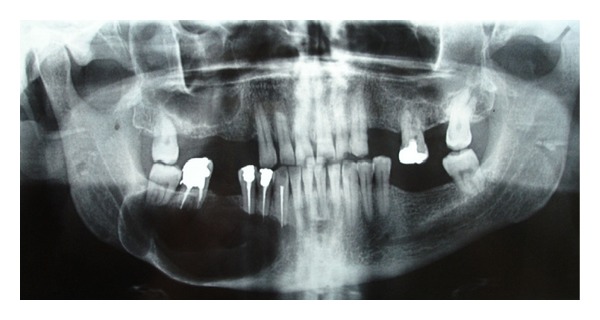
Panoramic radiograph; 2 weeks after operation.
